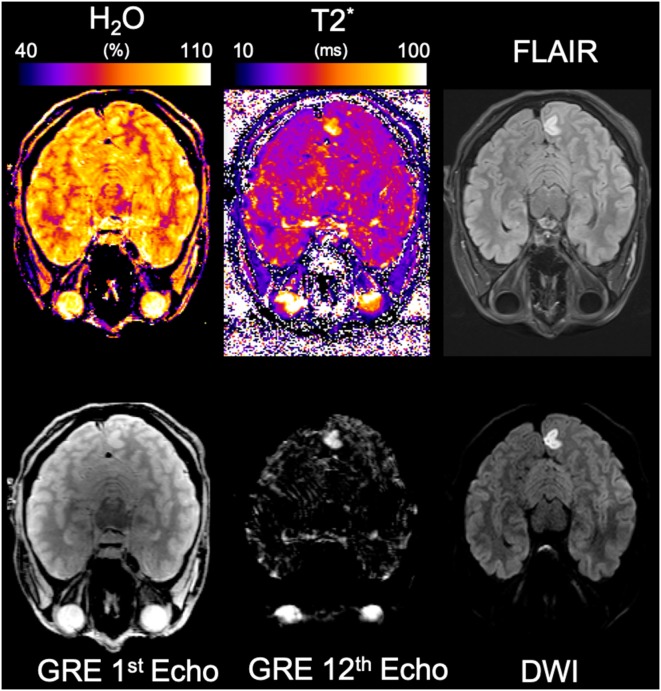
Results from the stroke patient. The same representative slice from the lesioned region is shown in each panel. The panels shown are, from left to right, top to bottom, water content, ${\text{T}}_{2}^{*}$, FLAIR, GRE 1st echo, GRE last echo, and diffusion-weighted imaging.
